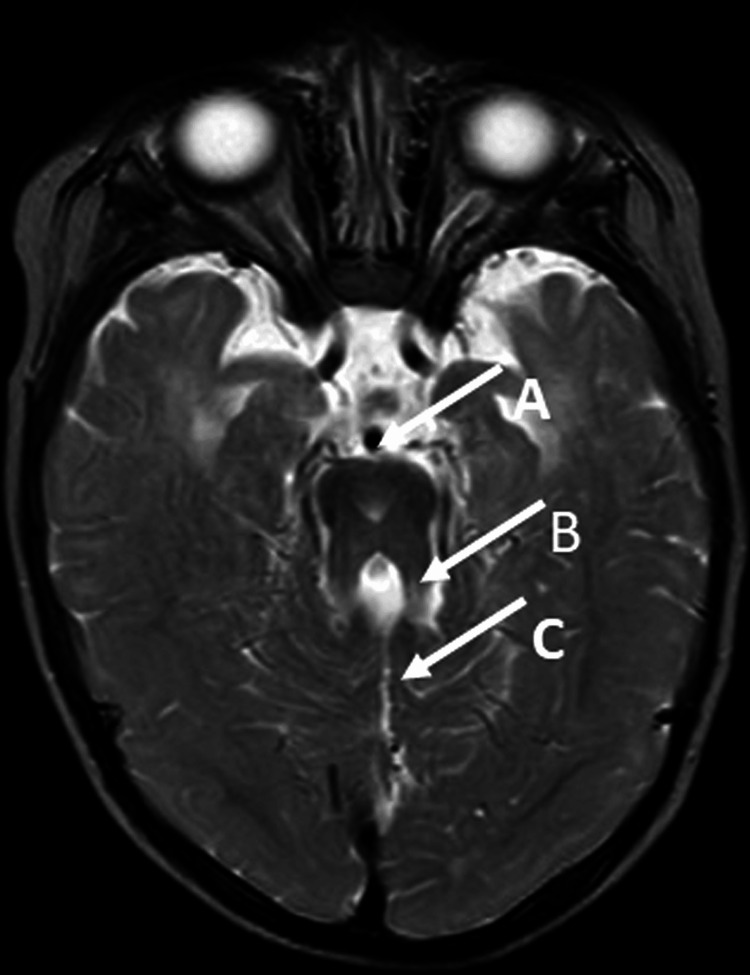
T2-weighted axial image on MRI of child 1 The MRI image was taken when she was 10 months old. The "Molar tooth sign" image is characterised by a deep posterior interpeduncular fossa (white arrow A), thickened and elongated superior cerebellar peduncles (white arrow B), and hypoplasia or agenesis of the cerebellar vermis (white arrow C). MRI: magnetic resonance imaging
